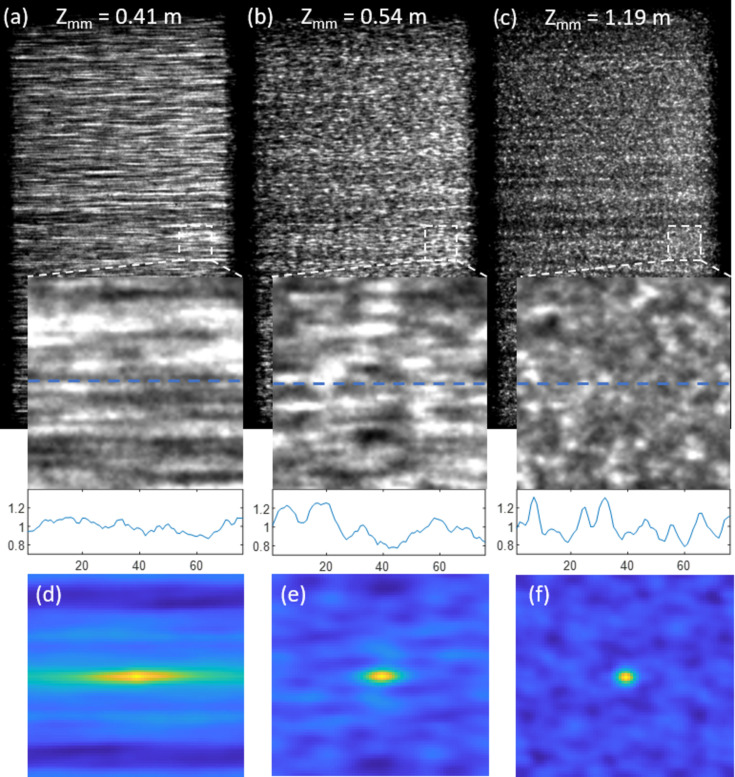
(*a*–*c*) Speckle images of an X-ray mirror at different mirror–modulator distances (*z*
